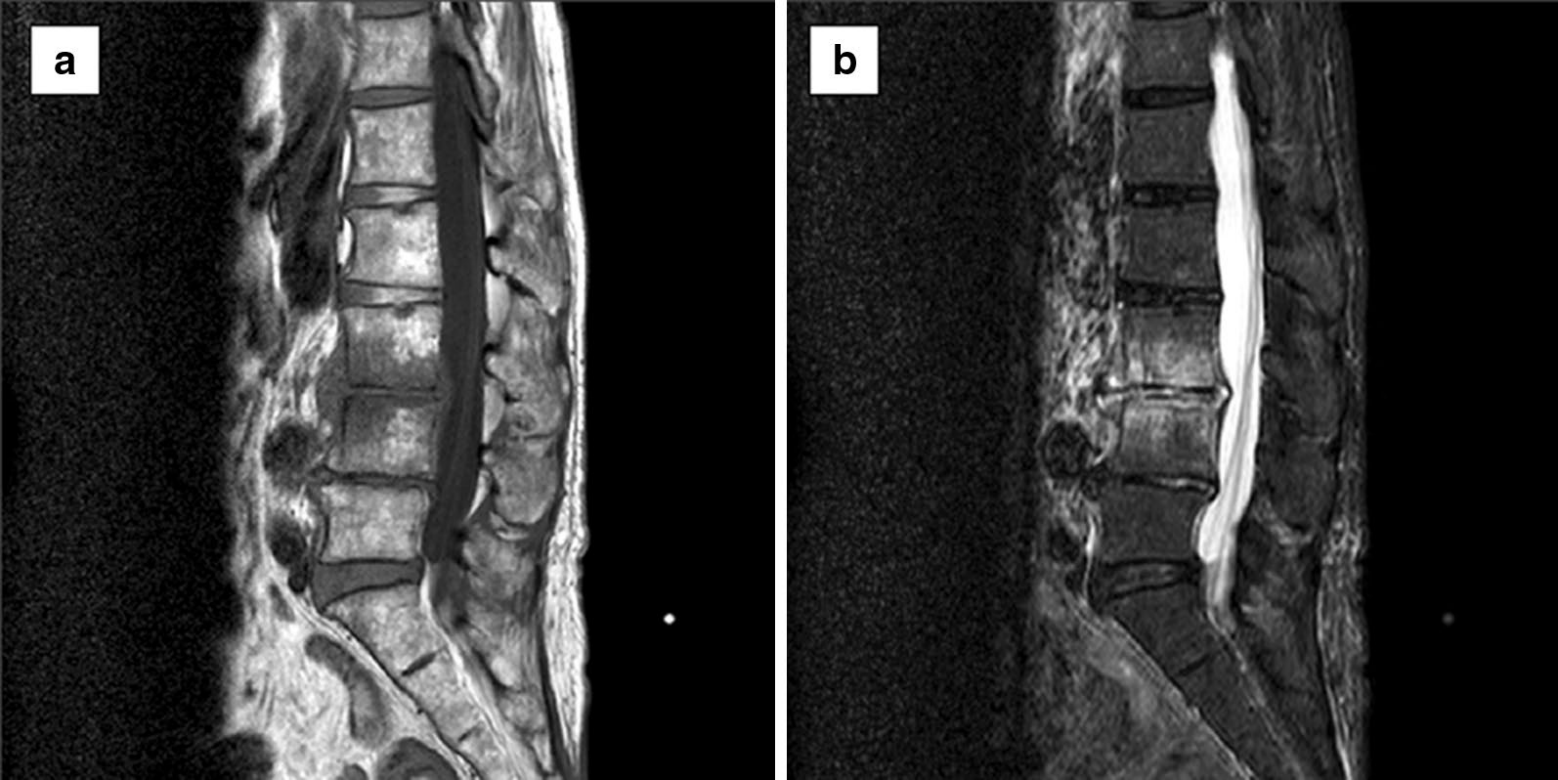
Magnetic resonance image of lumbar vertebrae obtained on the 10th hospital day. T1-weighted image (T1WI) and short T1 inversion recovery (STIR) T2 image (a, b), respectively. The lumbar sequence is linearized and the findings do not suggest a compression fracture, i.e. there is no dip-related transformation of the vertebral body. Vertebral bodies L3 and L4 have a low signal on T1WI and a high signal on STIR. The intervertebral disk is low and has a linear T2 high signal. We thus suspected this lesion to represent inflammation and/or degeneration
